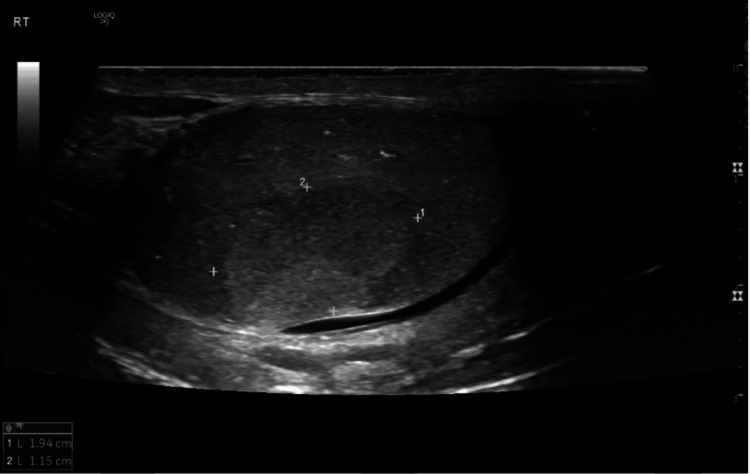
A right scrotal Doppler ultrasound U/S showed a well-defined vascularized heterogeneous mass measuring 2×1.2 cm in size, and there were multiple variable-sized hyperechoic areas with posterior shadowing.
